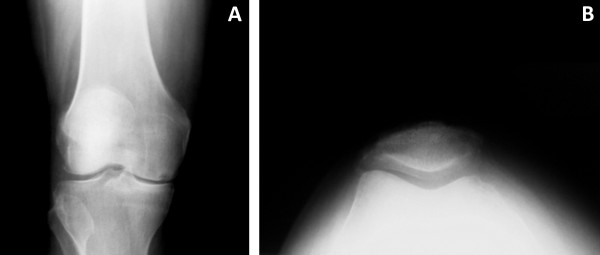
Pre-operative antero-posterior (A) and Merchant view (B) radiographs of a patient with medial compartment osteoarthritis treated with a metal-backed unicompartmental knee arthroplasty.
